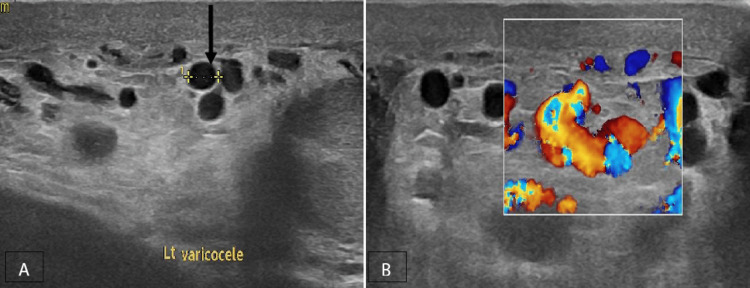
Varicocele A, B Grey-scale and colour Doppler images of the left side shows multiple dilated pampiniform plexus of veins with reflux (solid black arrow).
